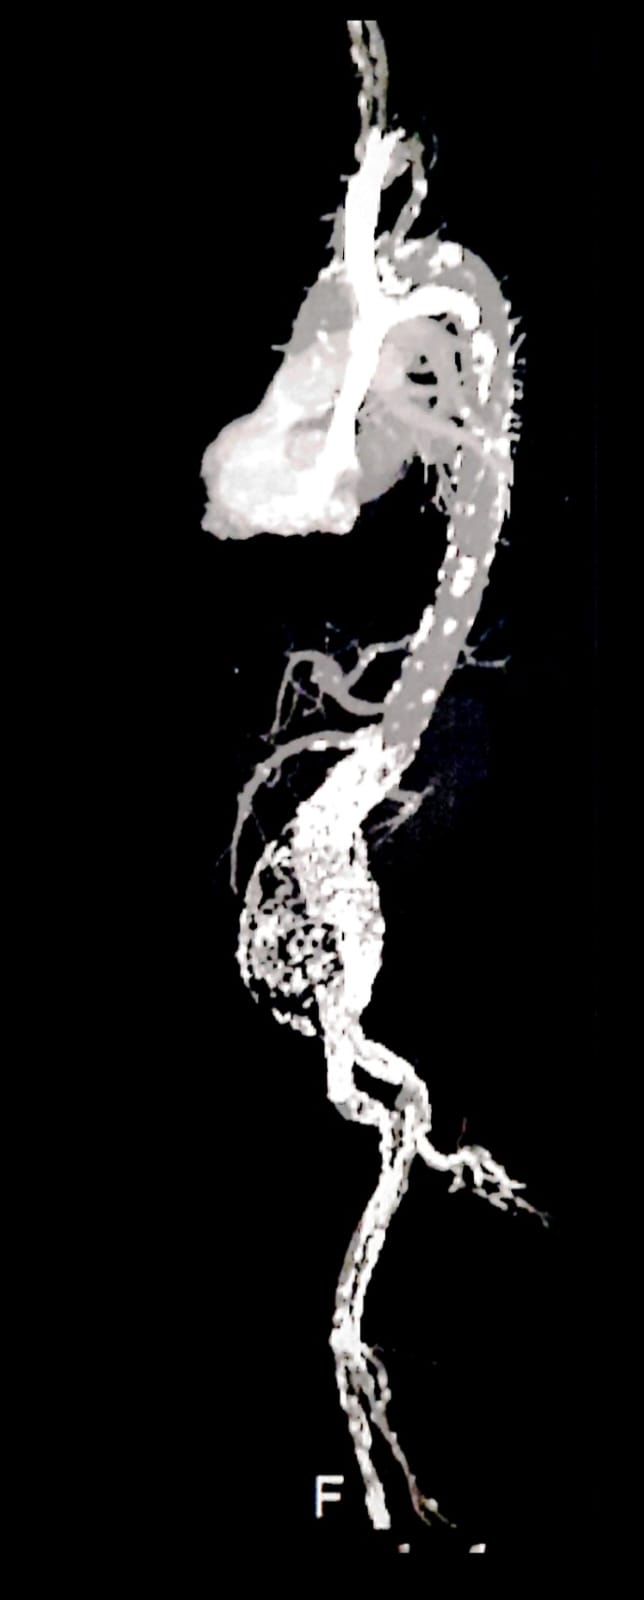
Control computed tomography angiography without contrast, showing the endograft structure intact and well positioned, in 2023.
